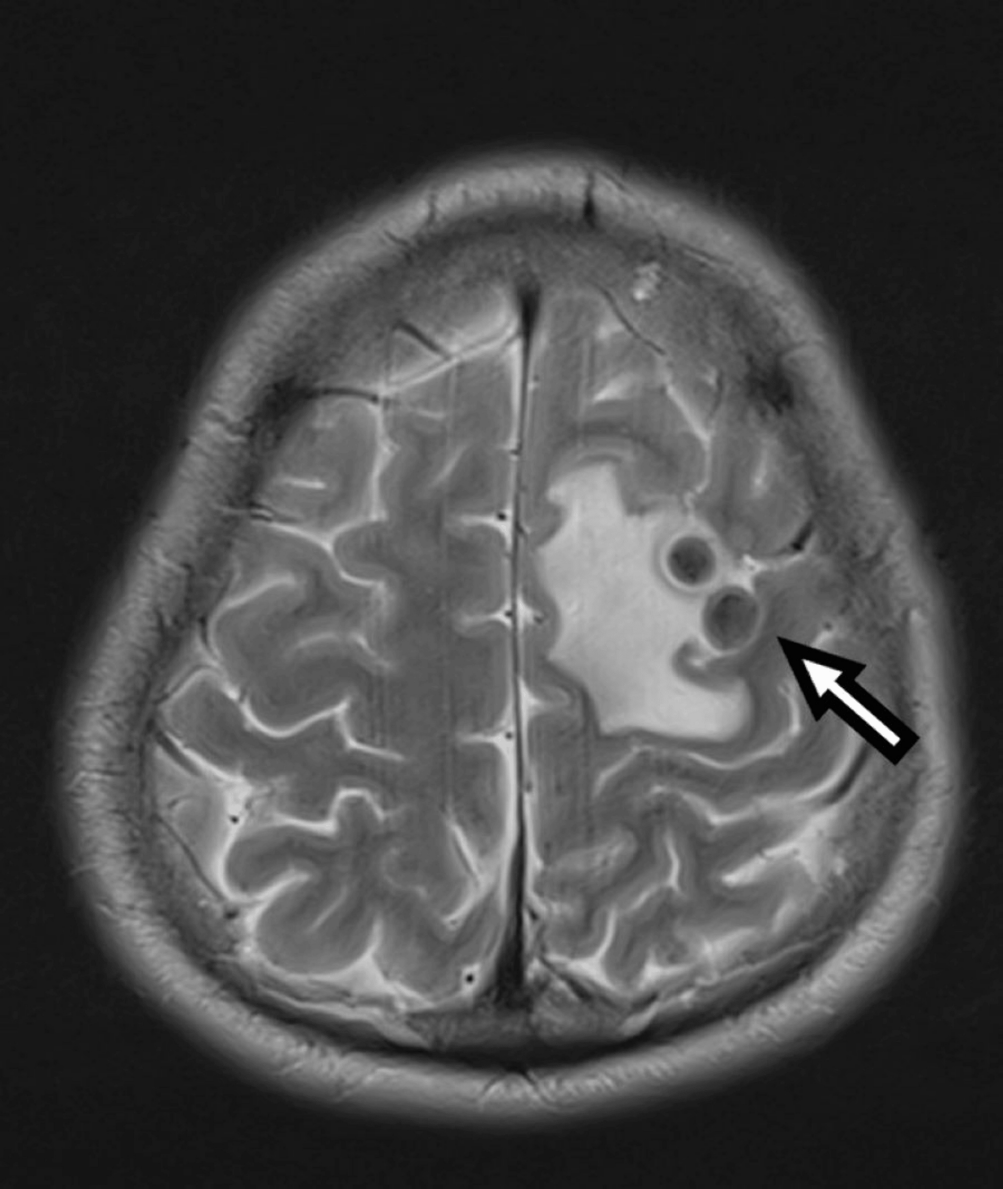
MRI of the brain, T2-weighted axial section, showing left frontal cysts with hypointense cystic wall with significant surrounding perilesional edema (white arrow). The left frontal cysts are similar to those in Figure 2.
